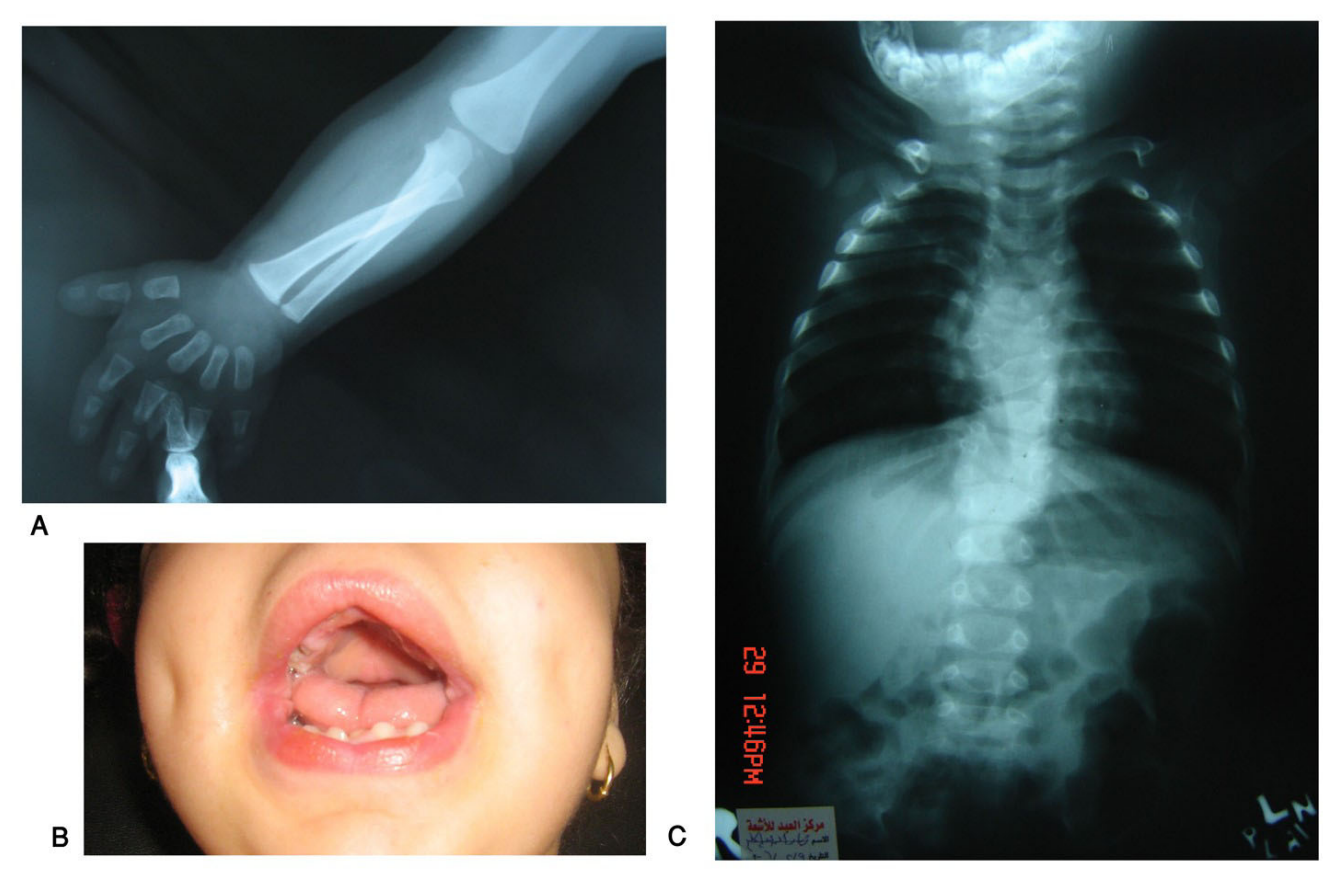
Autosomal recessive Robinow syndrome. X-ray upper limbs and hands showing mesomelic shortening and brachydactyly (A), gingival hyperplasia (B) and X-ray vertebrae showing hemivertebrae and vertebral fusion. (Limb Malformations & Skeletal Dysplasia Clinic, MSU, NRC).
